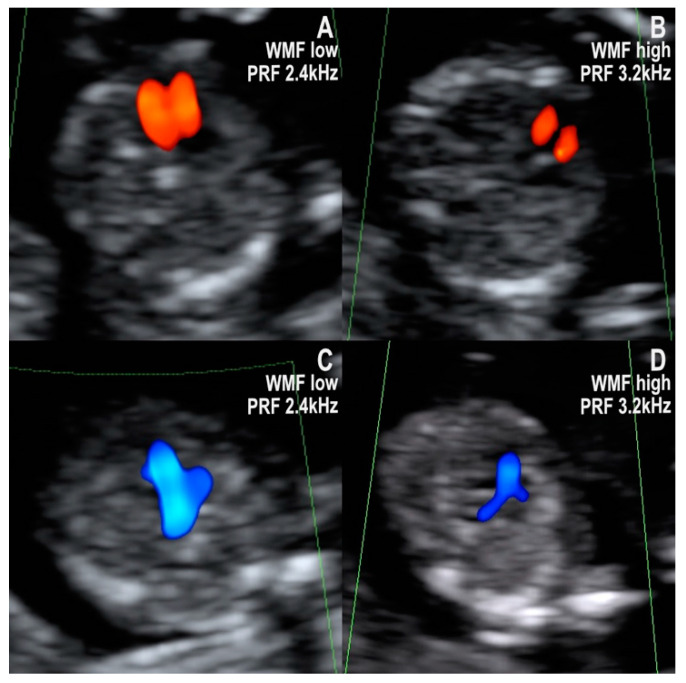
Color doppler flow patterns of the four-chamber, and three-vessel, and trachea views obtained using low and high PRF/WMF doppler settings in a normal 12–13-week fetus. (A): Low PRF/WMF doppler settings allow better visualization of the size and filling of the ventricles in the four-chamber view. (B): High PRF/WMF doppler settings are preferred to verify the distinct filling of the ventricles. (C): Low PRF/WMF doppler settings offer adequate visualization of the ductal and aortic arches. (D): High PRF/WMF doppler settings can impair the visualization of the aorta.
